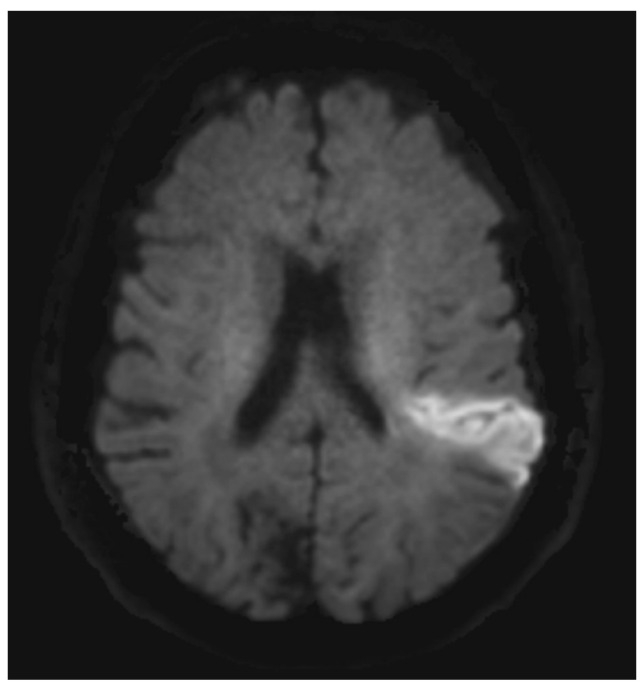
Diffusion-weighted imaging (DWI). Case of cardioembolic infarct in left hemisphere (bright area).
